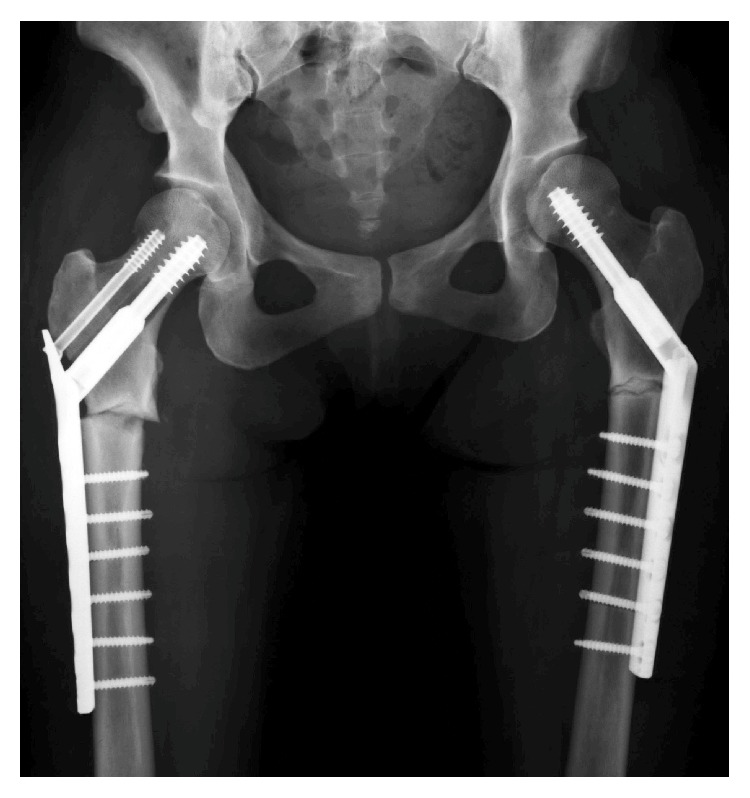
Radiograph after open reduction of the fractures with a plate and screws.
